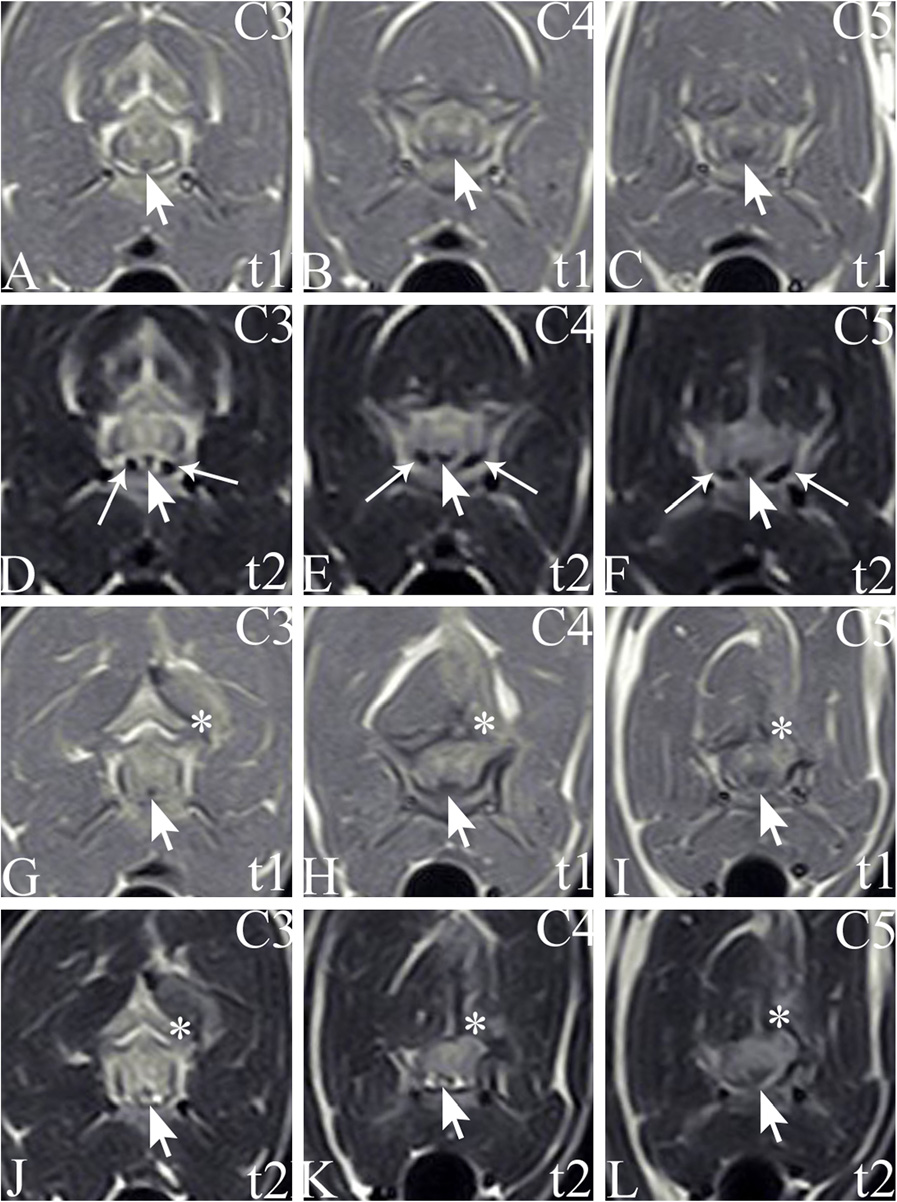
Transversal planes of magnetic resonance images before surgery (A, B, C, D, E, F) and after surgery (G, H, I, J, K, L). The symbols in the right upper corner encode images taken at the level of the third cervical vertebra (C3), the fourth cervical vertebra (C4) and the fifth cervical vertebra (C5). Intradural extramedullary/extradural hypointensity (pointer arrows) in T1W (t1) and T2W (t2) is causing ventrodorsal spinal cord flattening and compression. The asterisk shows post operative scar tissue, the arrows show the ventral sinuses.
